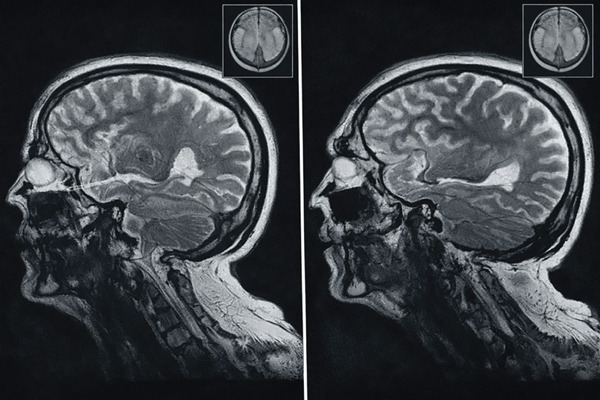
T1‐weighted sagittal MRI of the brain showing mild tonsillar herniation.
